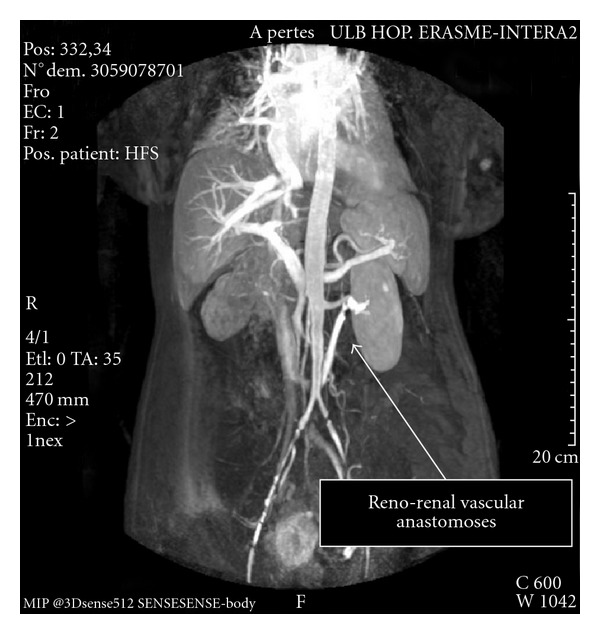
MRI angiography image after kidney transplantation in orthotopic position.
